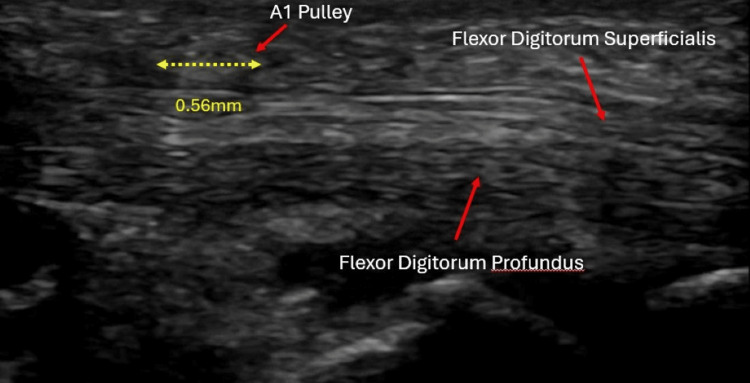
Follow-up ultrasound demonstrating decreased A1 pulley thickening (measured at approximately 0.56 mm) with improved visualization of the flexor tendons.
